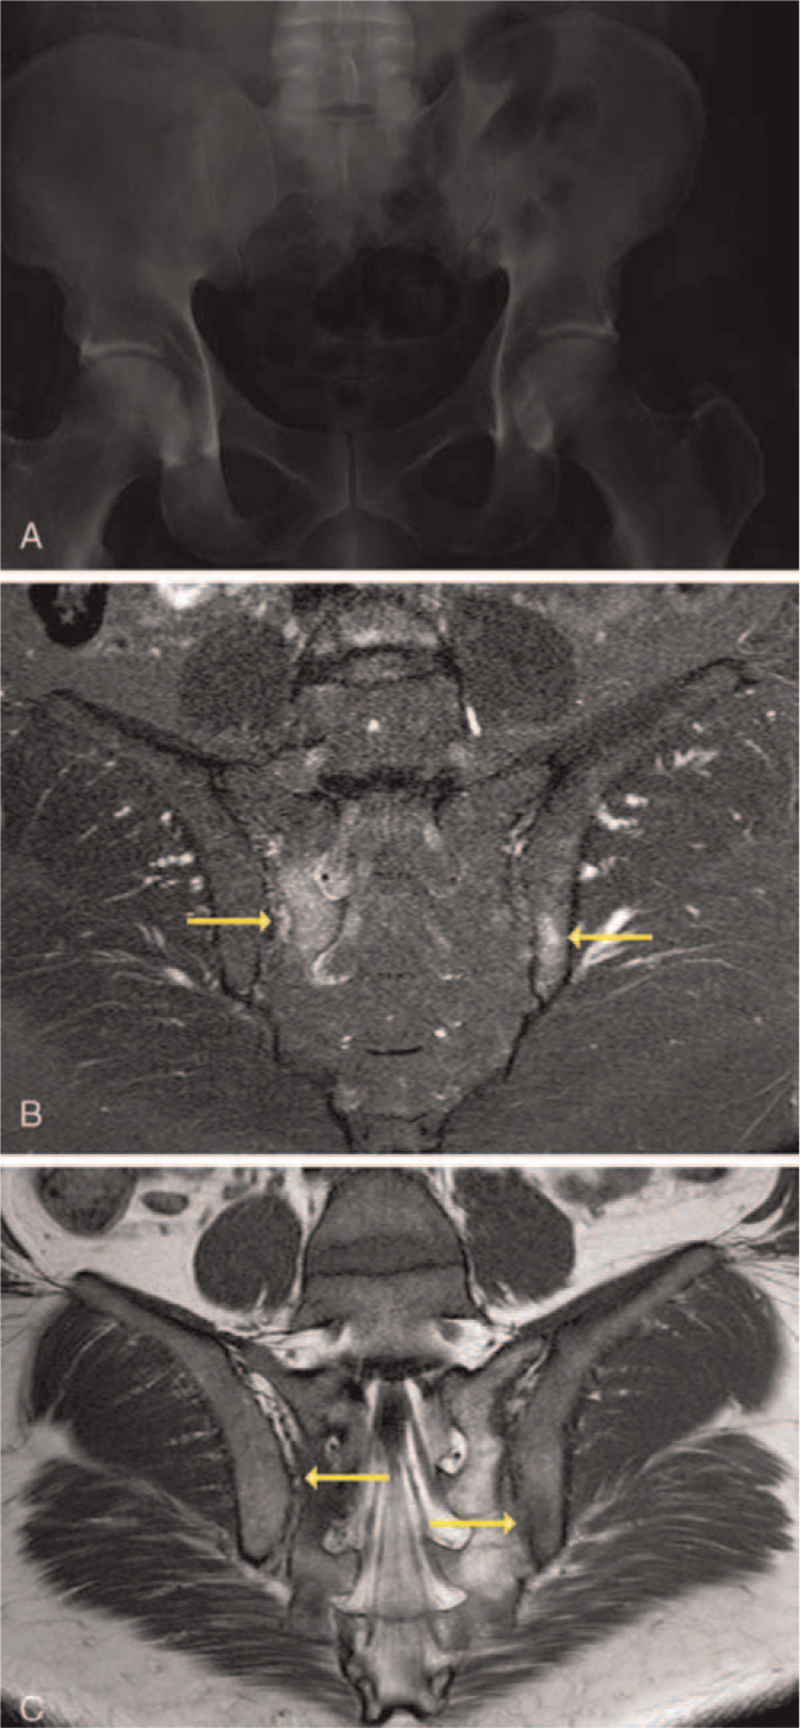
MRI plays an important role in the diagnosis of nr-axSpA. Imaging plays an important role in the diagnosis of nr-axSpA. In patients with nr-axSpA, X-ray images may be completely normal as these images do not allow detection of early signs of axial inflammation.^[49]^ The use of MRI of SIJs enables detection of osteitis (undetectable by X-ray) before radiographic sacroiliitis appears.^[49–51]^ (A) Shown is an X-ray image of a pelvis in a 45-year-old African-American patient with a 3-month history of low back and ankle pain. Pain wakes him at night and gets worse with prolonged sitting. The patient is HLA-B27-negative with a CRP level of 8.6 mg/dL. (B) Shown is an image of a STIR image showing high intensity edema (yellow arrows) on the sacral side of the right SIJ and iliac side of the left SIJ in the same patient. (C) Shown is a T1-weighted image with hypointensity areas showing edema with erosion (yellow arrows) on the sacral side of the right SIJ and iliac side of the left SIJ in the same patient. Patient images were provided courtesy of Dr Marina Magrey. axSpA = axial spondyloarthritis, CRP = C-reactive protein, MRI = magnetic resonance imaging, nr-axSpA = nonradiographic axial spondyloarthritis, SIJ = sacroiliac joint, STIR = short tau inversion recovery.
